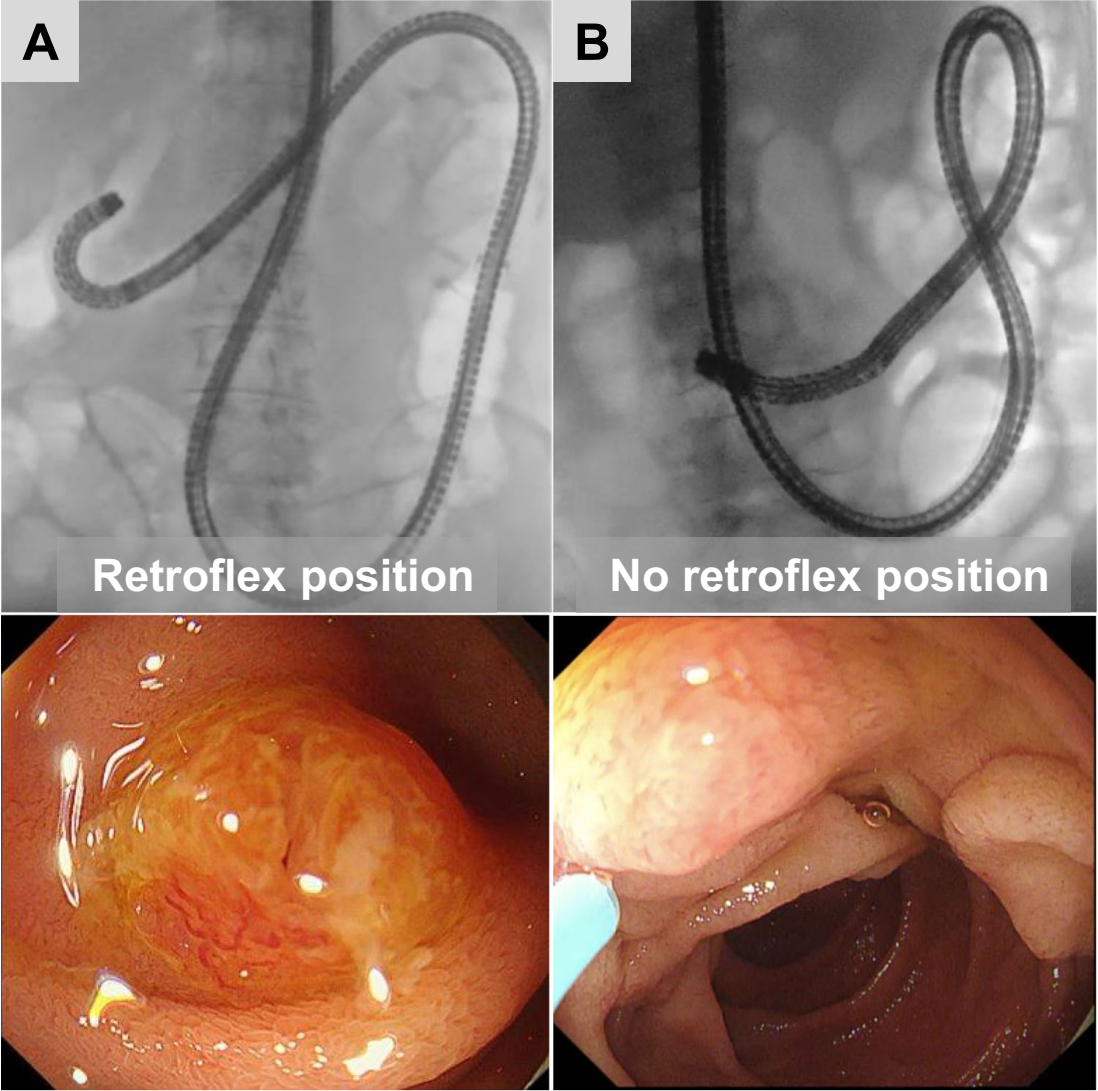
(A) Retroflex position. (B) No retroflex position. The upper panels show two radiographic images, and the lower panels show two endoscopic images.
